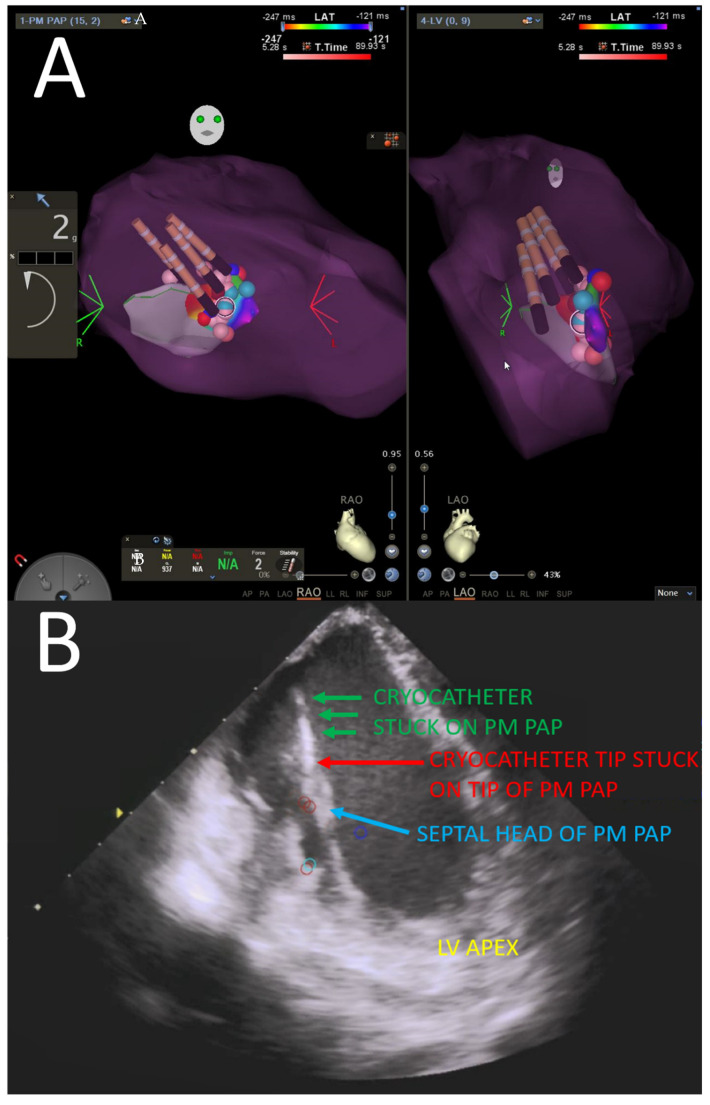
Papillary muscle ablation utilizing cryo-energy and intracardiac echocardiography. (A) Electroanatomic map highlighting cryo-energy lesions to a papillary muscle. (B) Intracardiac echocardiography is a useful adjunct to confirm good contact and stability of catheter to papillary muscle during ablation. PM PAP: posteromedial papillary muscle, LV: left ventricle.
